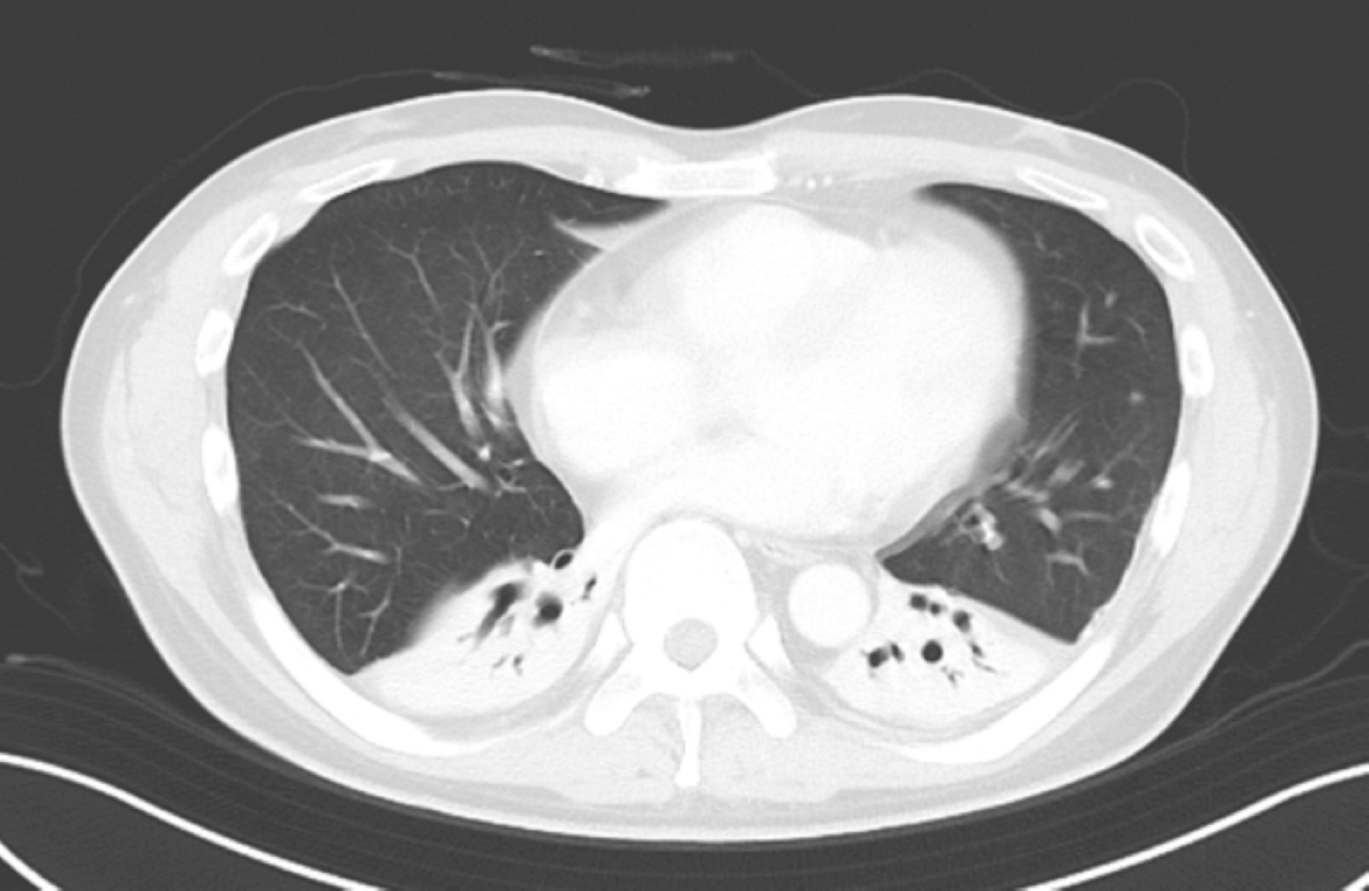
Chest CT on the ninth sick day Follow-up axial chest CT image on day 9 in a patient with ARDS secondary to *Salmonella septicemia*. The image reveals near-complete resolution of the previous bilateral ground-glass opacities, with preservation of normal bronchovascular markings. There is persistent atelectasis or homogeneous opacities with air bronchograms extending internally in the bilateral dependent zones. Pleural effusions have completely resolved. This radiological improvement is consistent with clinical recovery. CT: computed tomography, ARDS: acute respiratory distress syndrome
